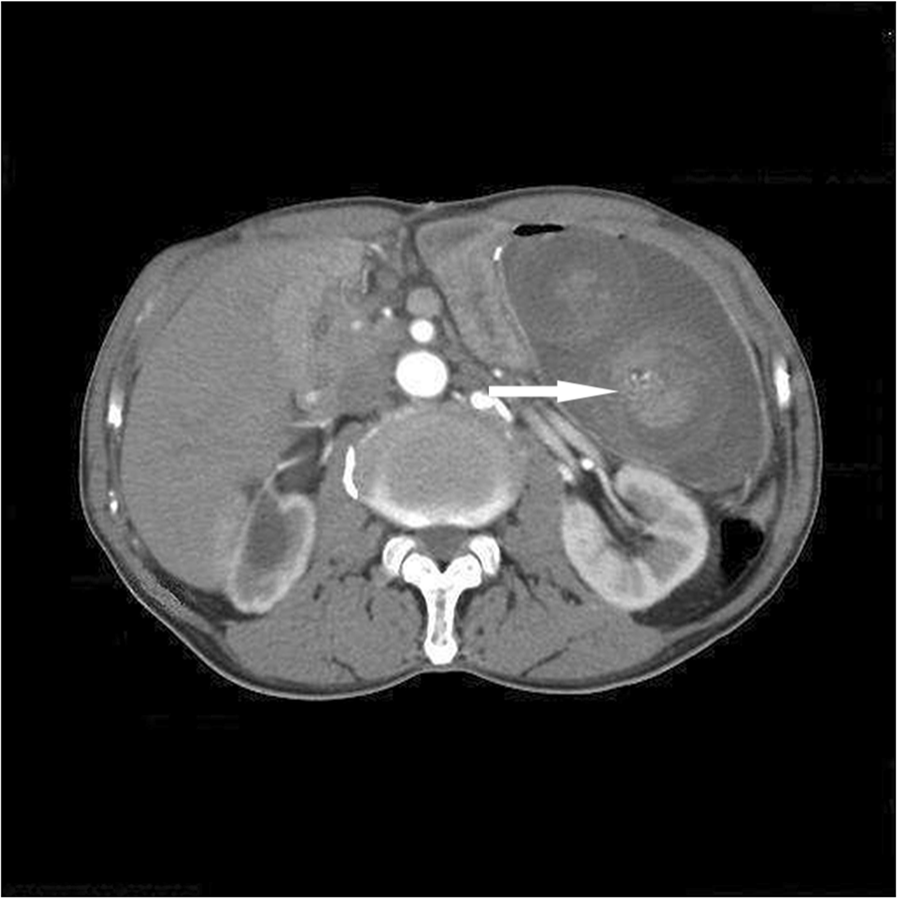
Abdominal CT scan. The CT-scan reveals dilated stomach with bowel loops (Arrow: jejunogastric intussusception)
